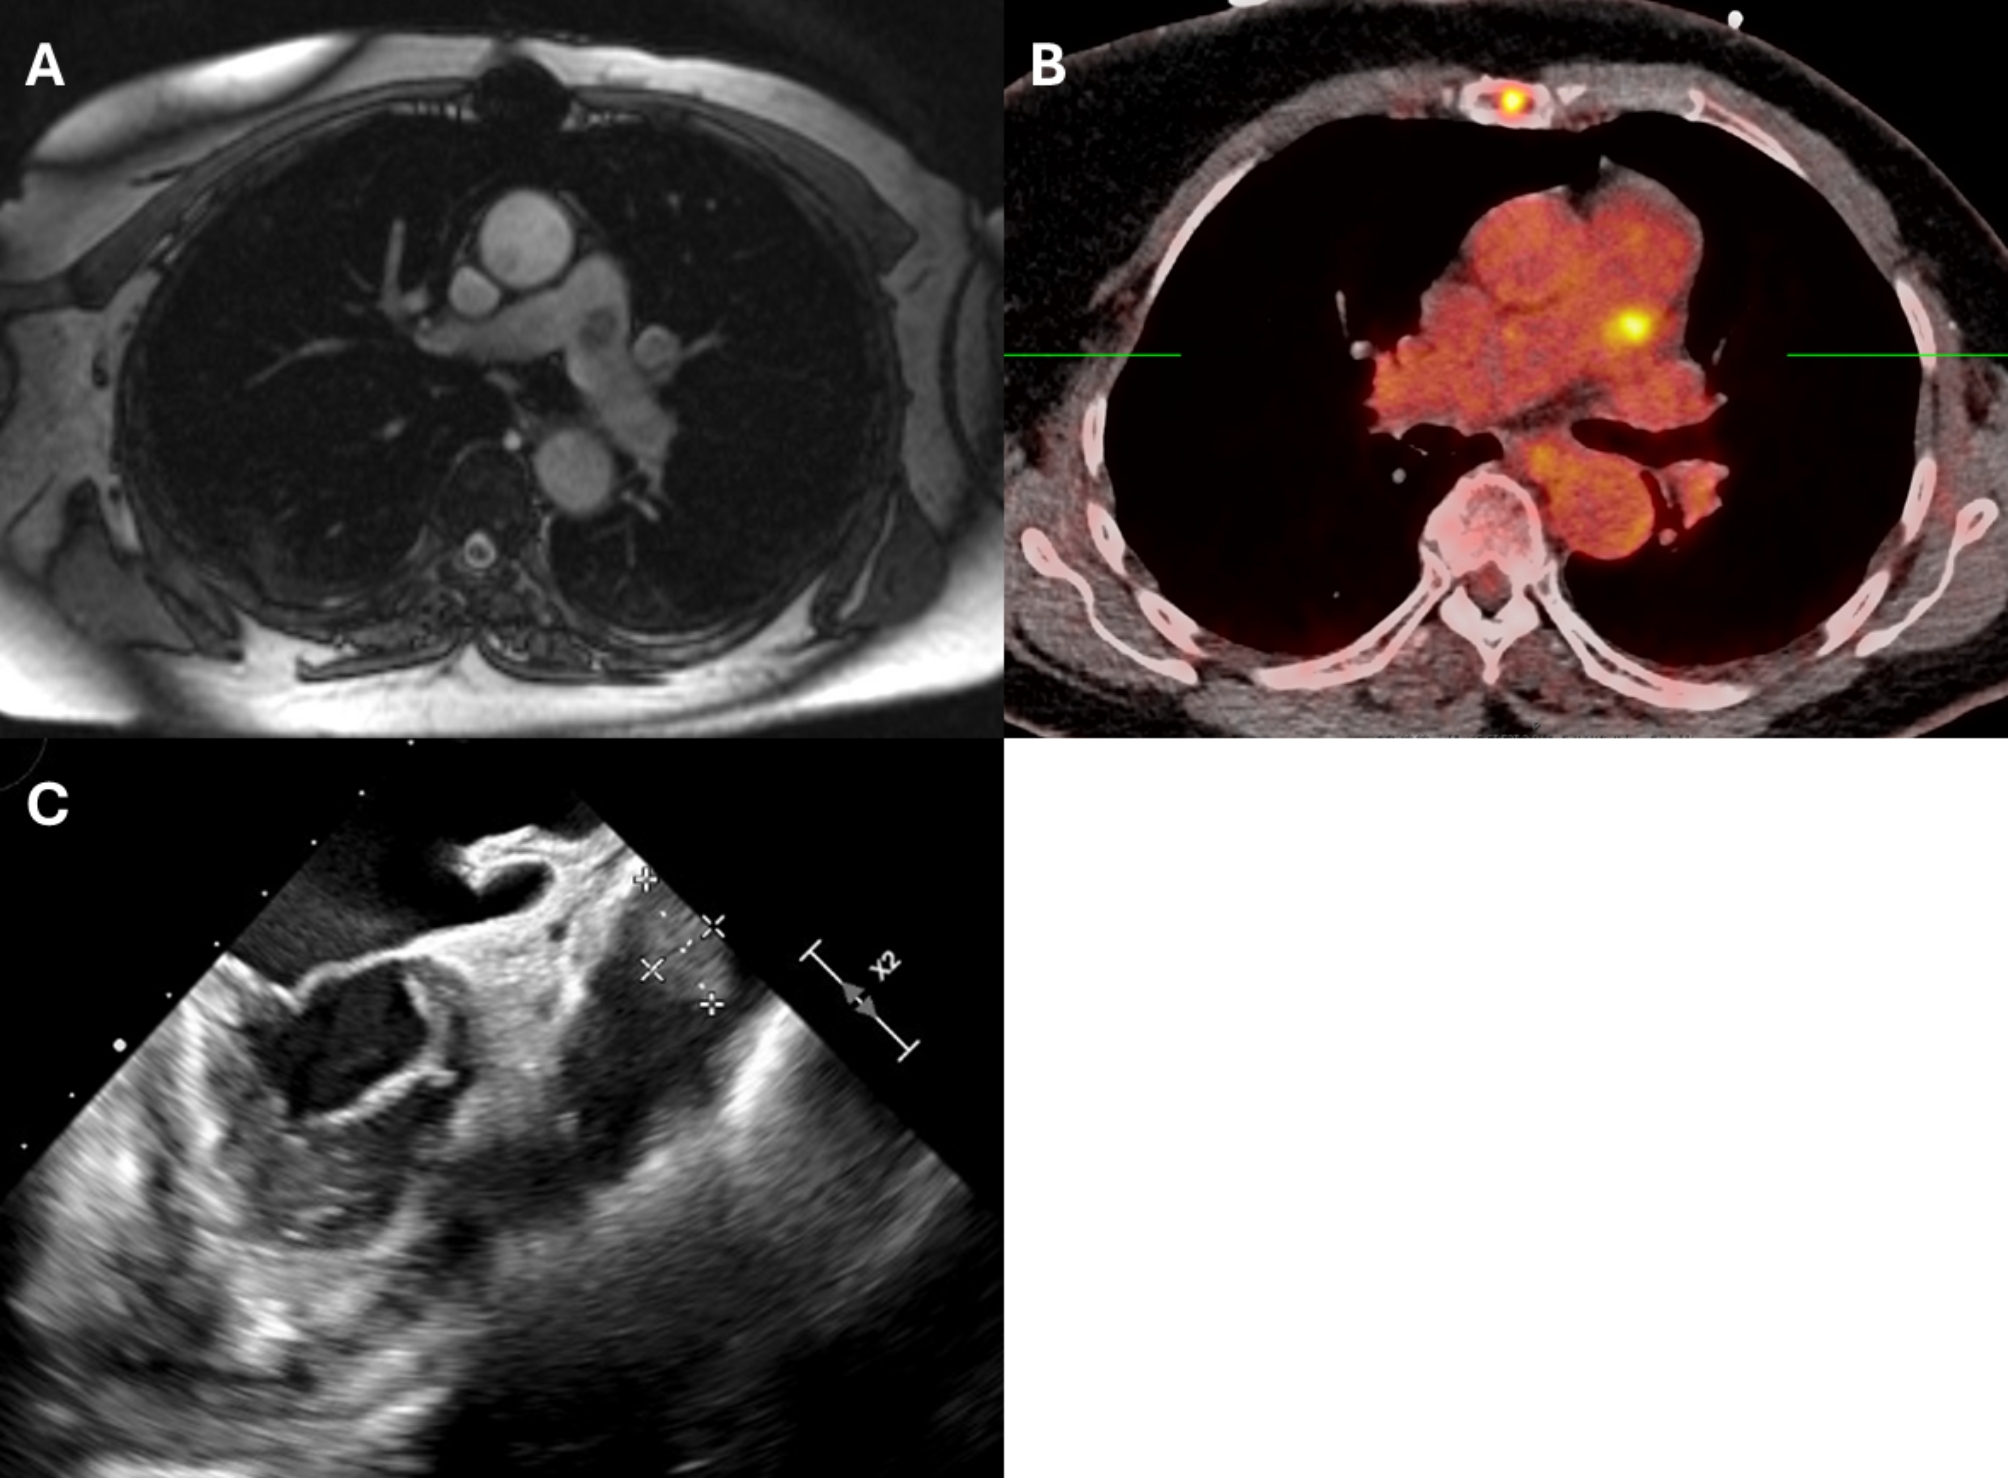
Diagnostic imaging of pulmonary artery mass shown by (A) cardiac magnetic resonance imaging; (B) PET computed tomography; (C) transesophageal echocardiography
